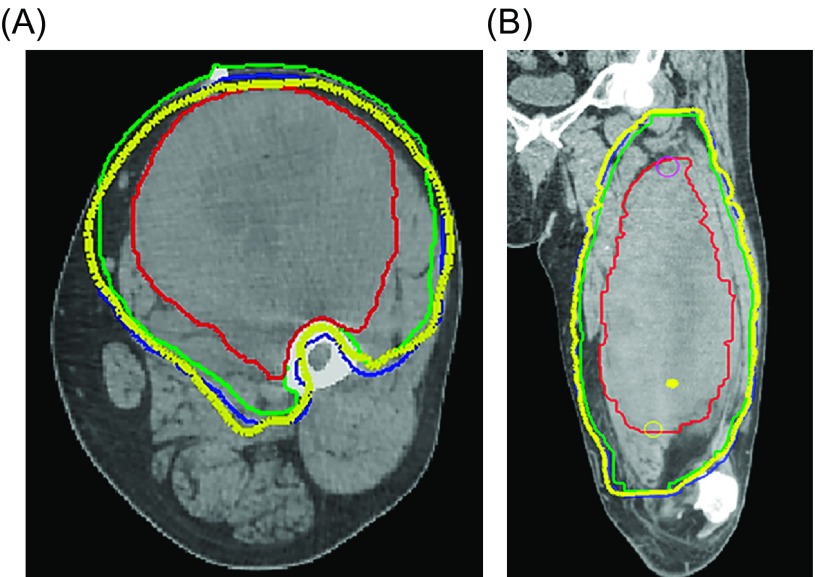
Preoperative radiotherapy planning volumes for the patient in Figure 1 are shown on axial (A) and coronal (B) CT images. The Gross Tumor Volume (GTV) is demonstrated by the solid red contour; Clinical Target Volume (CTV) is demonstrated by the green solid contour; Planning Target Volume (PTV) is shown by the blue solid contour; and the thick yellow line represents the prescribed radiotherapy dose volume. Note that intensity-modulated radiotherapy (IMRT) was used to adequately encompass the radiotherapy target volume while avoiding the bone by sculpting the high dose volume around the femoral cortex for protection purposes (A), while also accounting for the peritumoral edema surrounding the lesion (B) which was demonstrated on the coronal fat-saturated T2 post-gadolinium image in Figures 1C and 1D.
